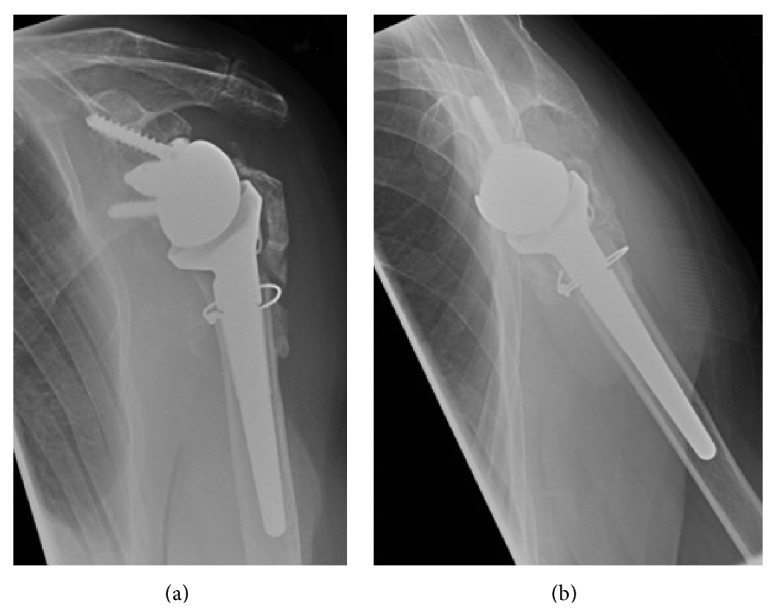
Final AP (a) and lateral (b) radiographs of RSA at 6 months. Default surgical technique is to place wire around proximal humerus to prevent its fracturing during preparation and press fit of humeral component.
